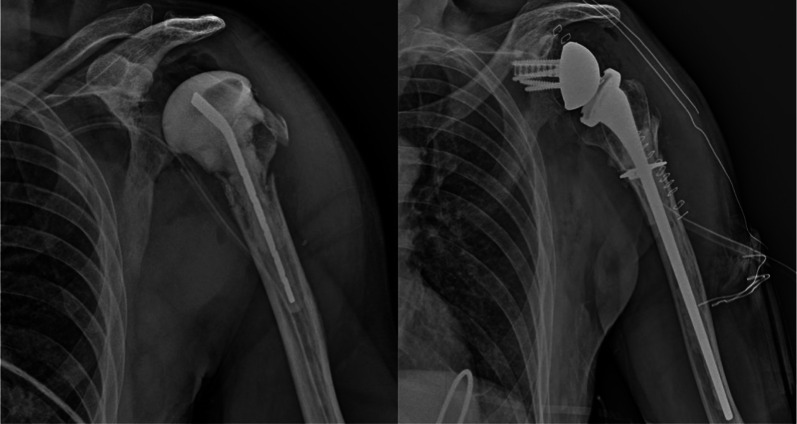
Preoperative radiograph with an antibiotic-loaded spacer and postoperative radiograph of a revision shoulder prosthesis with allograft reconstruction
